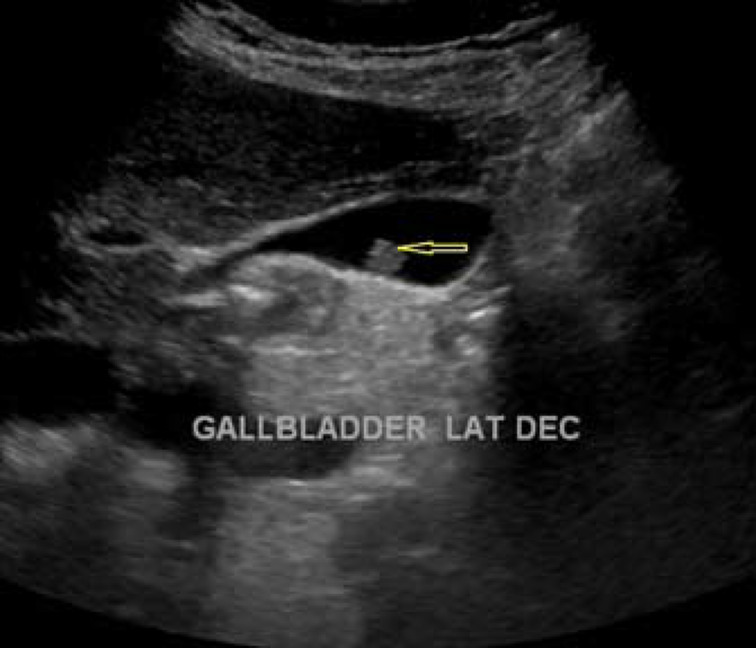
Ultrasound image showing a gallbladder polyp (yellow arrow)
